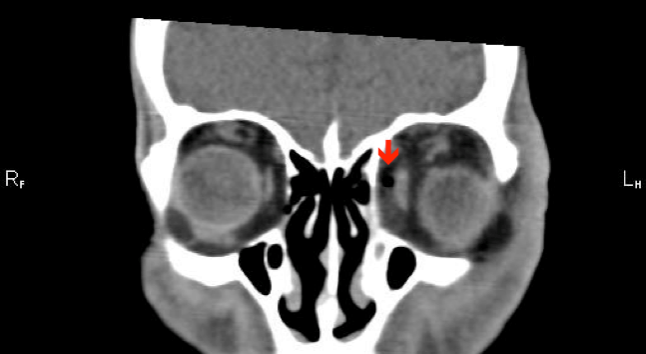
Coronal section of MRI scan showing air locule
